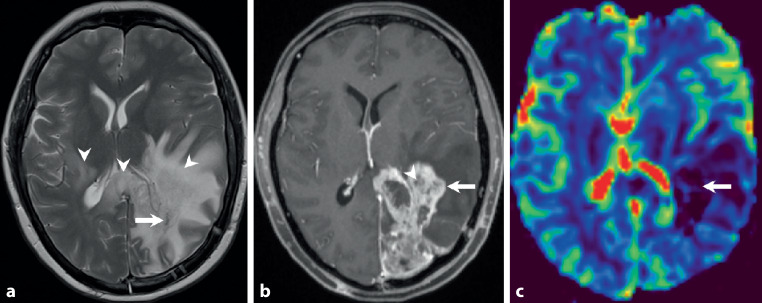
t = 7 weeks. On-going combined radiotherapy and chemotherapy. The hyperintense signal intensity changes have markedly increased on axial T2-weighted images (a, *arrowheads*). No signs of a solid residual tumor are on display (a, *arrow*). On contrast-enhanced T1-weighted images (b) a thick patchy rim of contrast enhancement along the margins of the tumor resection is visible (*arrow*) interleaved with smaller areas of regressive changes (*arrowhead*). On axial cerebral blood volume (CBV) maps of perfusion-weighted MR images (c) no hyperperfusion is visible in the areas of increased contrast enhancement (*arrow*)
